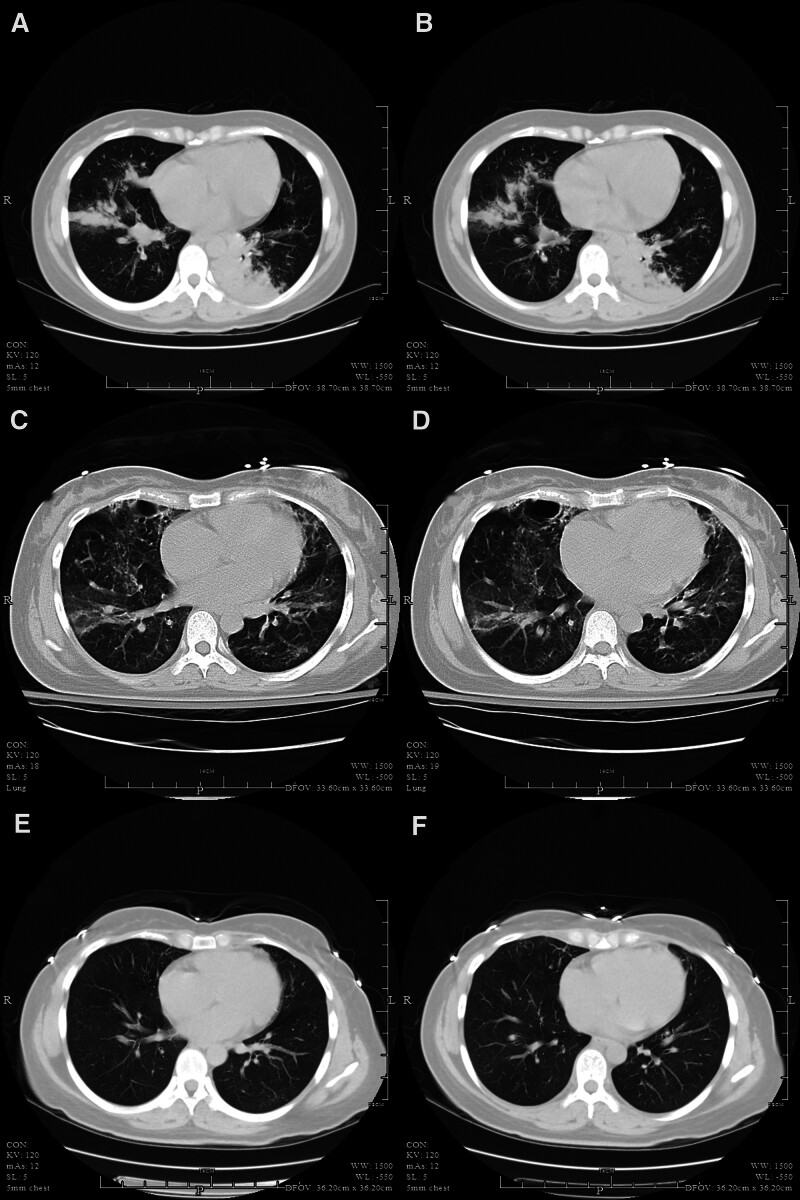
Imaging (A, B) March 3, 2023 chest CT showed multiple infiltrates in both lungs. (C, D) On April 2, 2023, chest CT showed slightly less multiple infiltration in both lungs. (E, F) July 29, 2023 chest CT showed multiple infiltrates in both lungs, which were fewer than before. CT = computed tomography.
